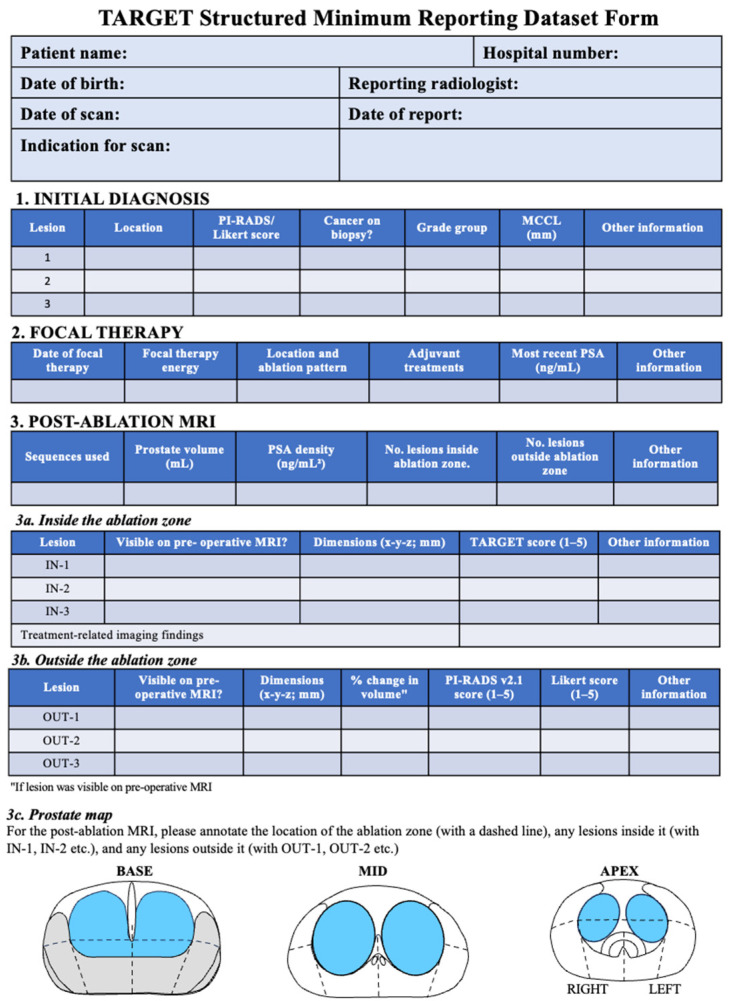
TARGET post-focal therapy MRI reporting data set. MCCL = maximum cancer core length; MRI = magnetic resonance imaging; PI-RADS = Prostate Imaging Reporting and Data System.
